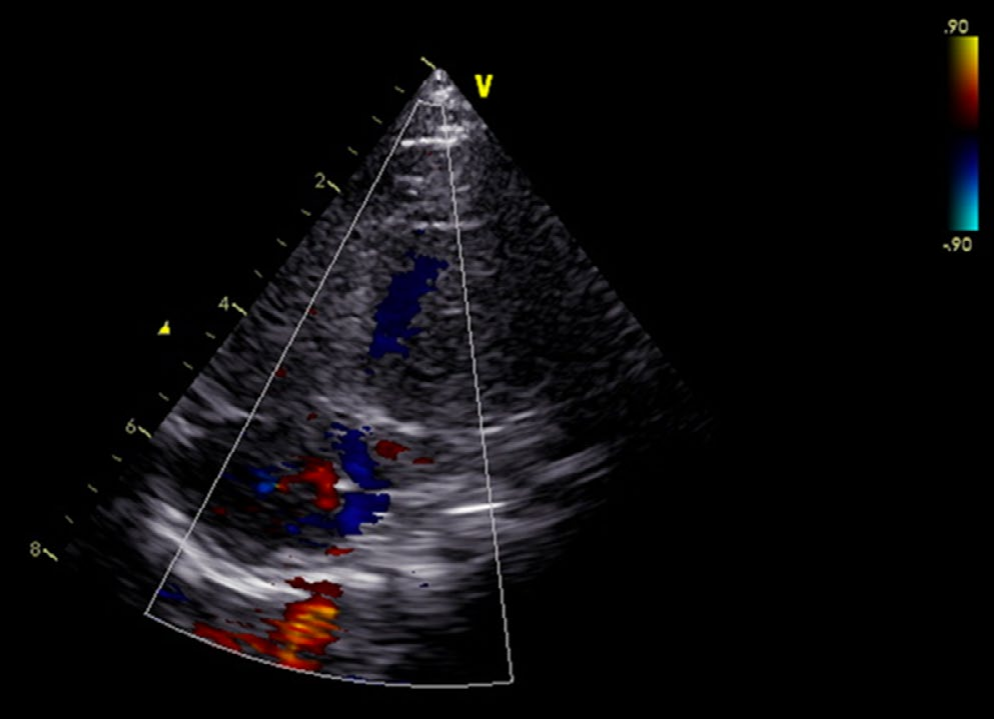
Postoperative echocardiography (six months later) demonstrating no atrial septal defect and no pericardial effusion
